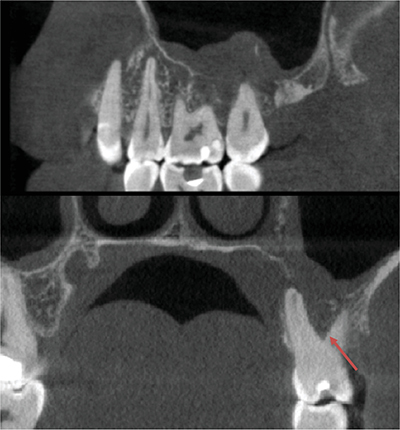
Computed tomography showing a large osteolytic area between left sinus and left upper molars (10 x10 cm (668 x 668 x 668)- 0,150 mm- 90 kV-8 mA 15,117s, ROMEXIS software).
